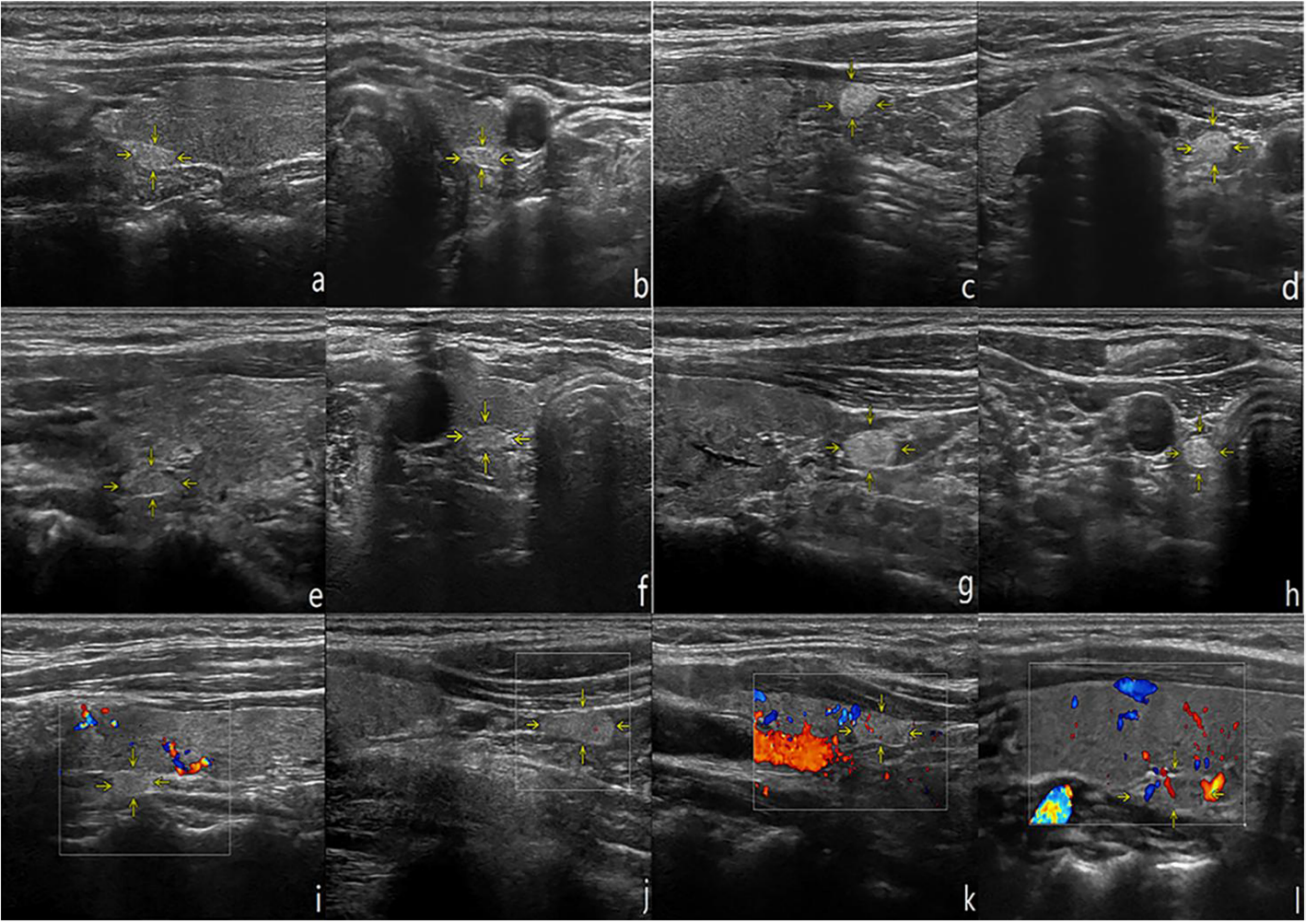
Routine Ultrasound Characteristics of normal PTGs. a-d: Longitudinal and transverse views of the left superior and left inferior PTGs, respectively. e-h: Longitudinal and transverse views of the right superior and right inferior PTGs, respectively. i-l: Internal blood flow signal of PTGs, in the order of grade 0 to grade III
